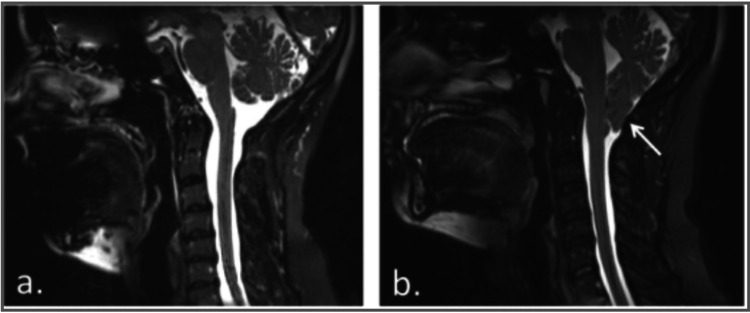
Sagittal T2-weighted MRI showing normal tonsillar position (a) and inferior cerebellar tonsillar herniation consistent with CM-I (b, arrow) Image reproduced from Open-i, National Library of Medicine (NIH) [22] (CC BY 4.0 Deed). MRI: magnetic resonance imaging; CM-I: Chiari malformation type I
